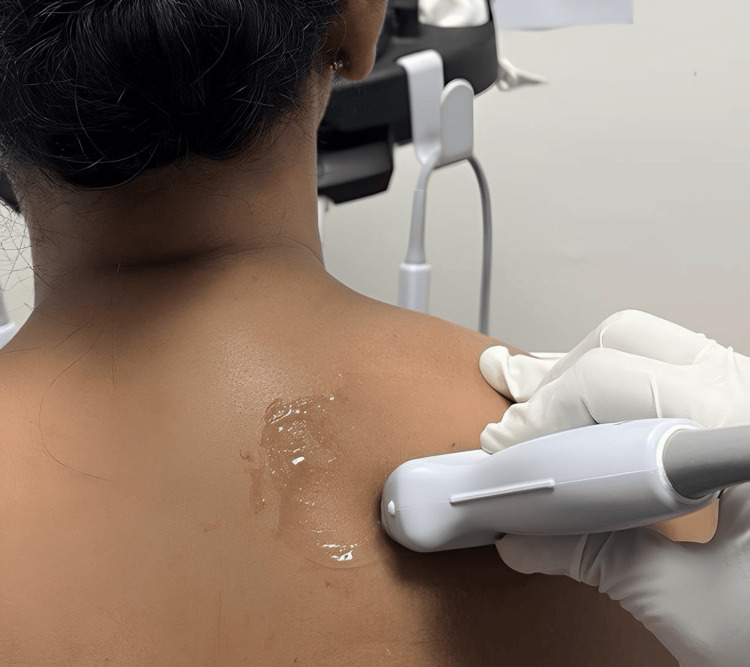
Ultrasound probe at the level of scapular spine in transverse view
